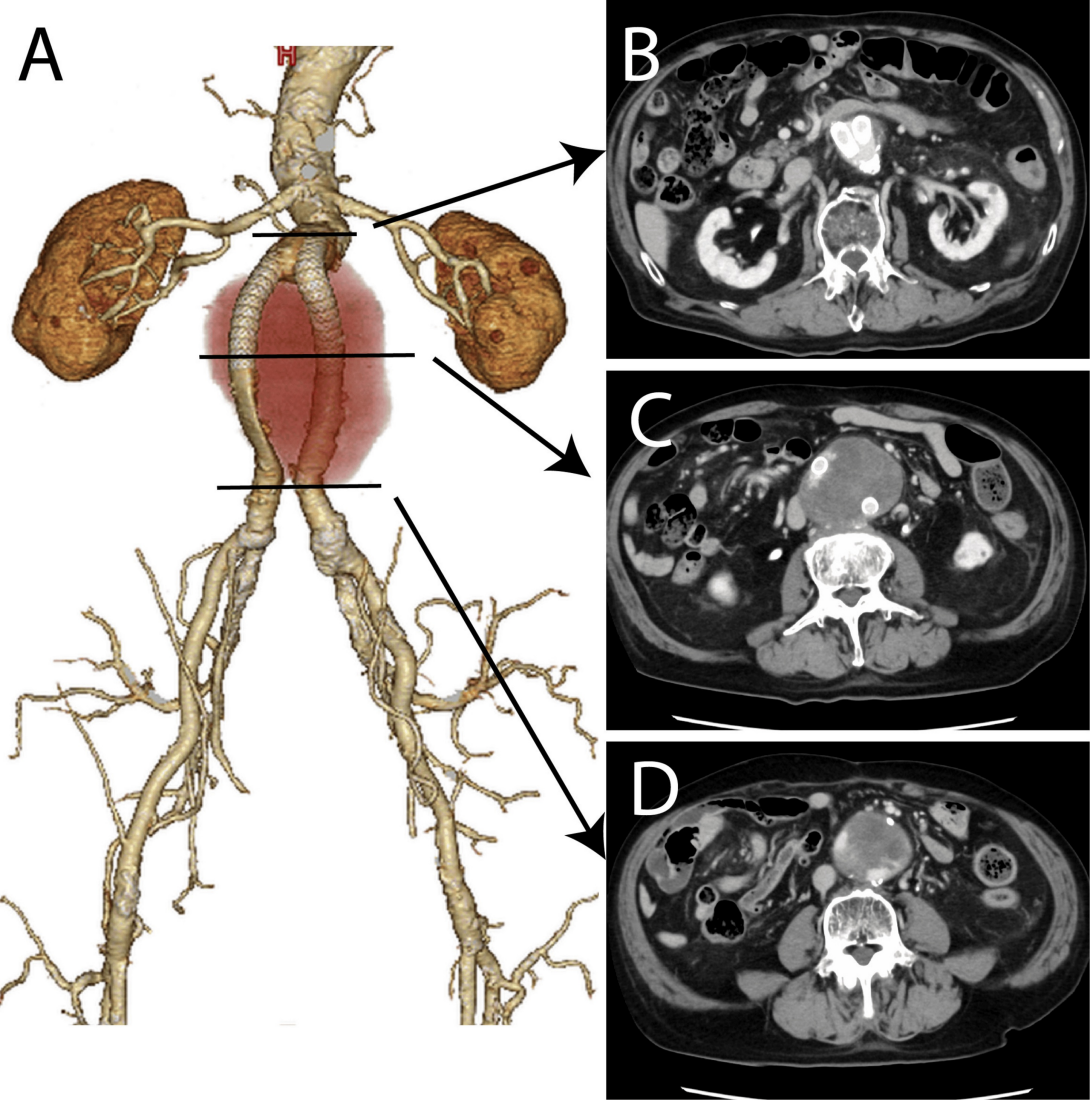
Contrast computed tomography image taken 37 years after artificial blood vessel replacement surgery in which a covered stent was placed inside the artificial blood vessel. (A) Volume rendering image. (B) A leak from an artificial blood vessel is visible at the proximal site. (C) A leak is visible at the end of the leg of the covered stent. (D) A leak from the artificial blood vessel is visible at the end of the artificial blood vessel.
